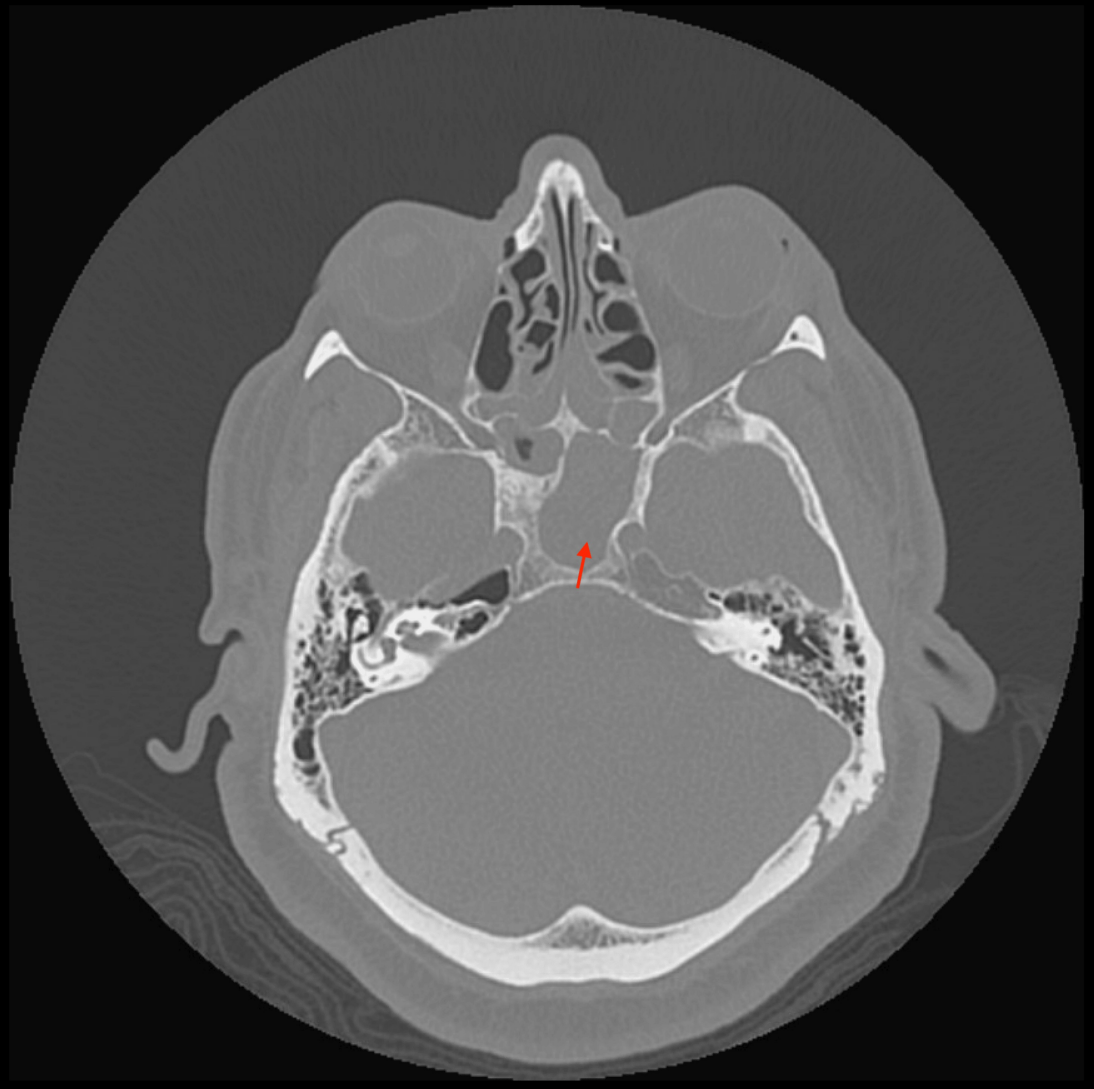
CT widening of the left sphenoid ostium, with thinning and sclerosis of the surrounding sphenoid sinus/clivus (arrow) CT: Computed tomography
